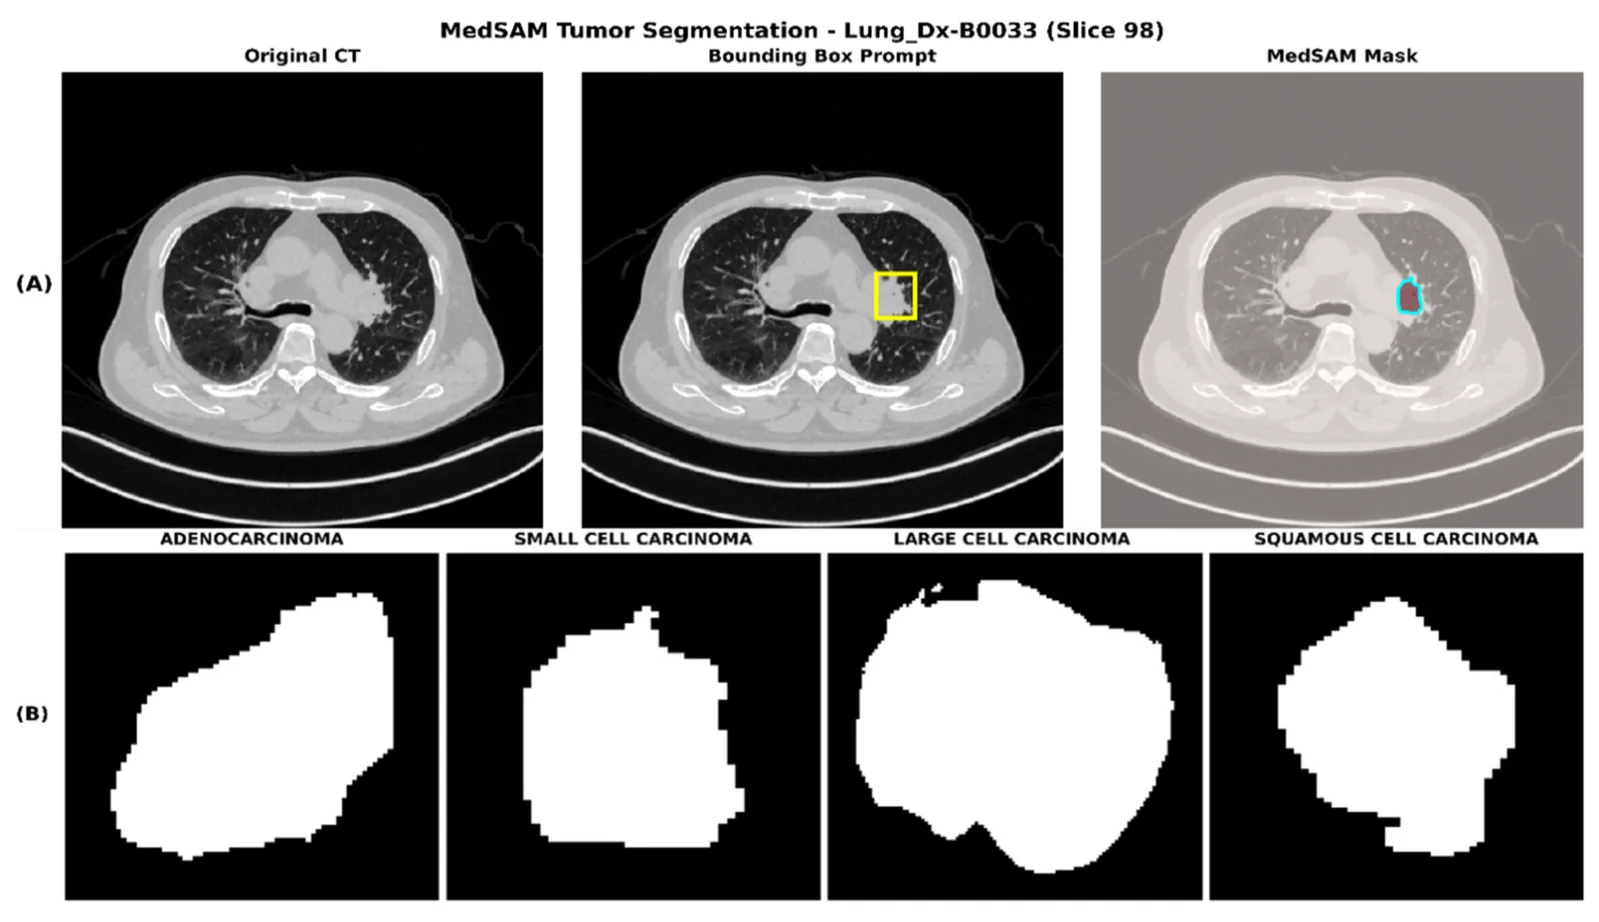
Representative stages of tumor segmentation: (A) original CT slice, bounding box overlay, MedSAM-generated tumor mask; (B) class-wise predicted masks.
